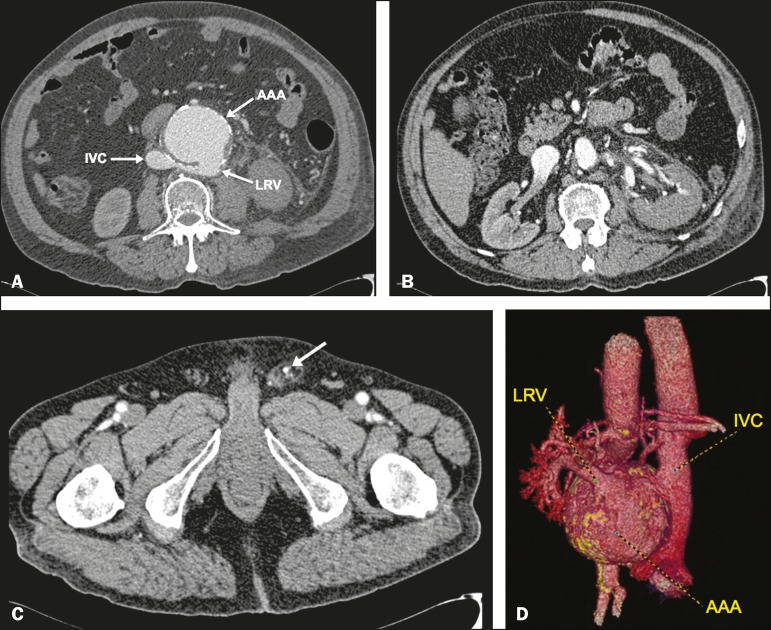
A-C: Contrast-enhanced axial CT slices showing equal
